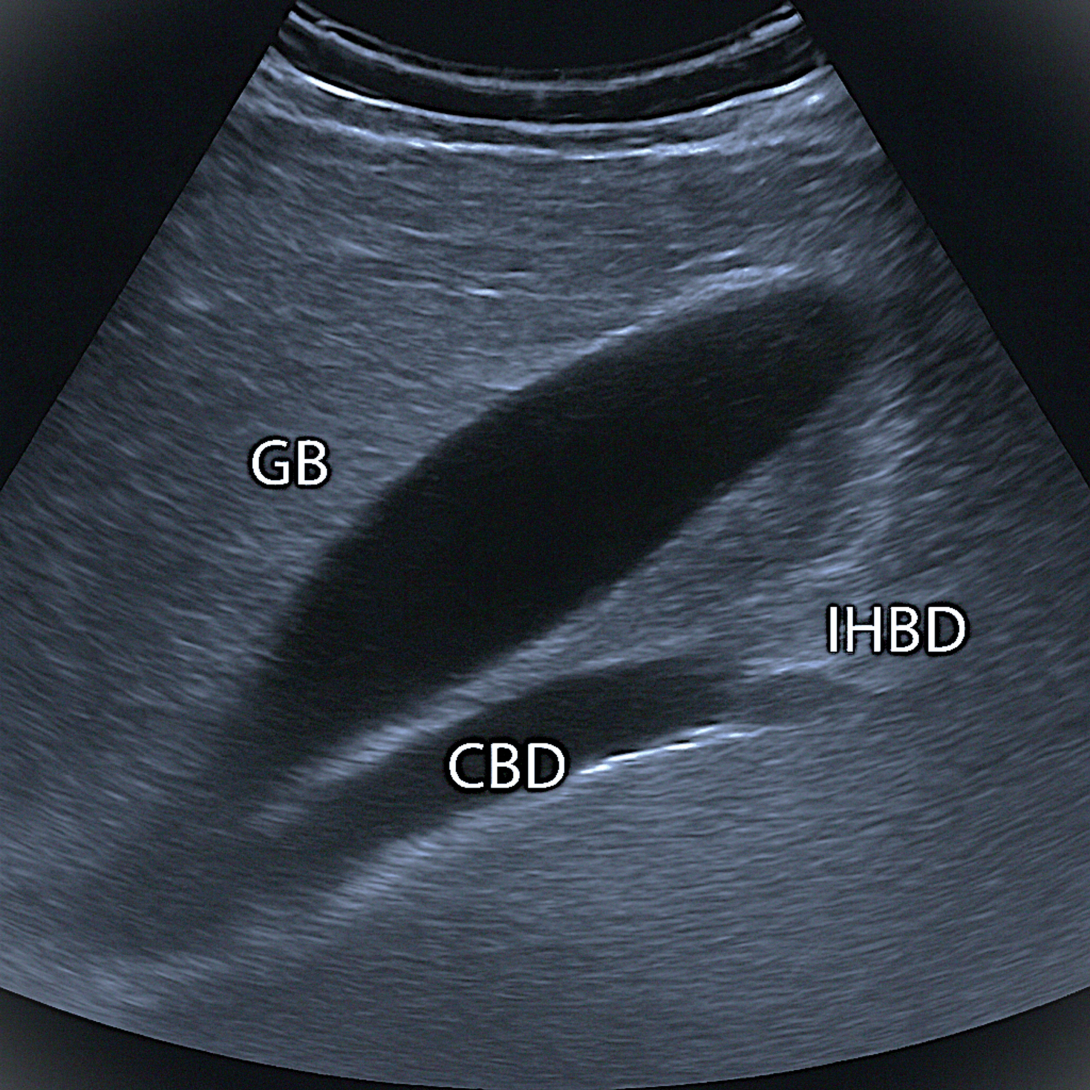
Ultrasound abdomen showing distended gallbladder and intrahepatic biliary dilatation GB: gallbladder; CBD: common bile duct; IHBD: intrahepatic biliary dilatation
